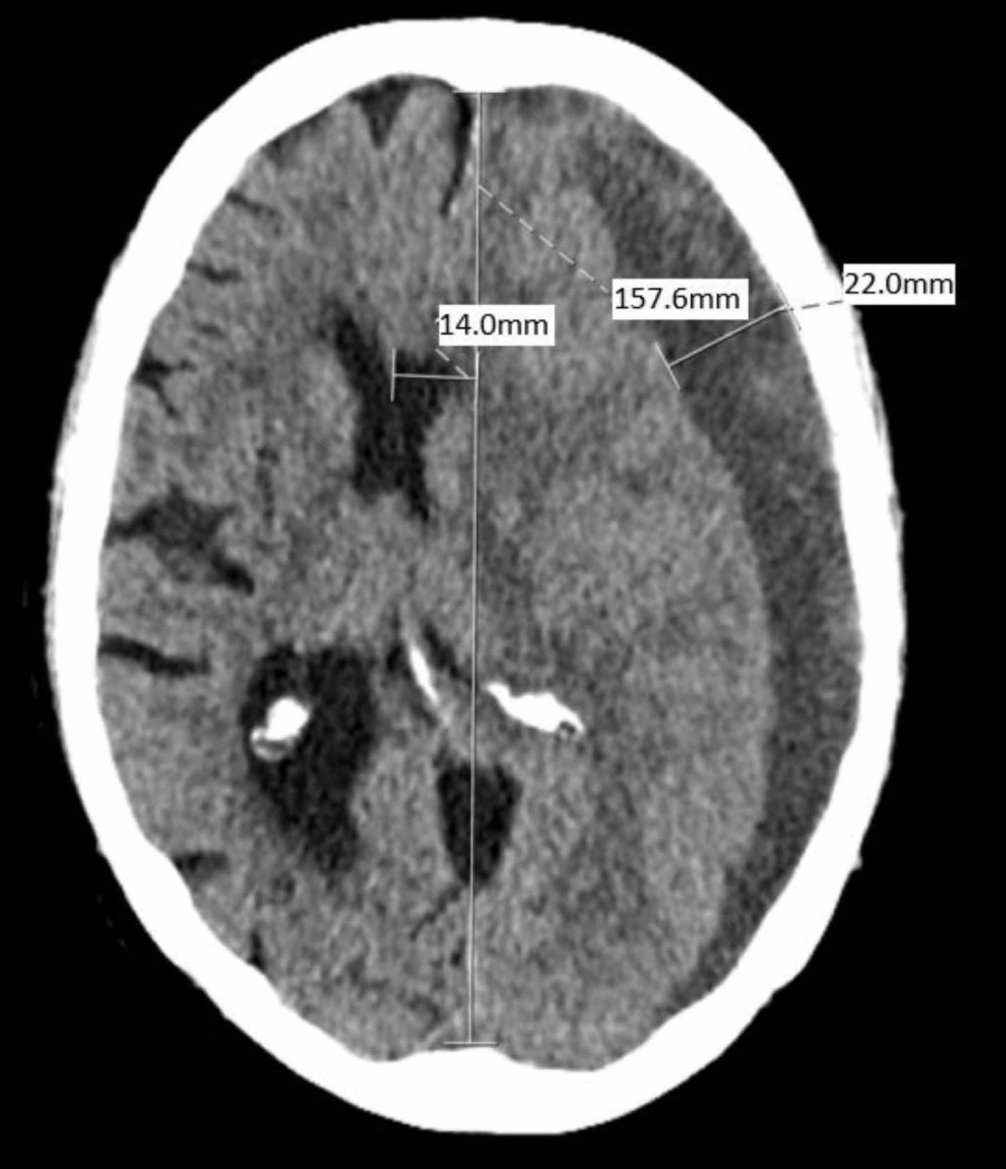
The patient’s presenting CT examination demonstrating a large, left-sided, crescent-shaped frontoparietal sSDH measuring 22 mm at the deepest point, with 14 mm of midline shift, left to right. The SDH has a mixed density, with components varying from hypodense to isodense compared to the brain. CT: computed tomography; sSDH: subacute subdural hematoma
